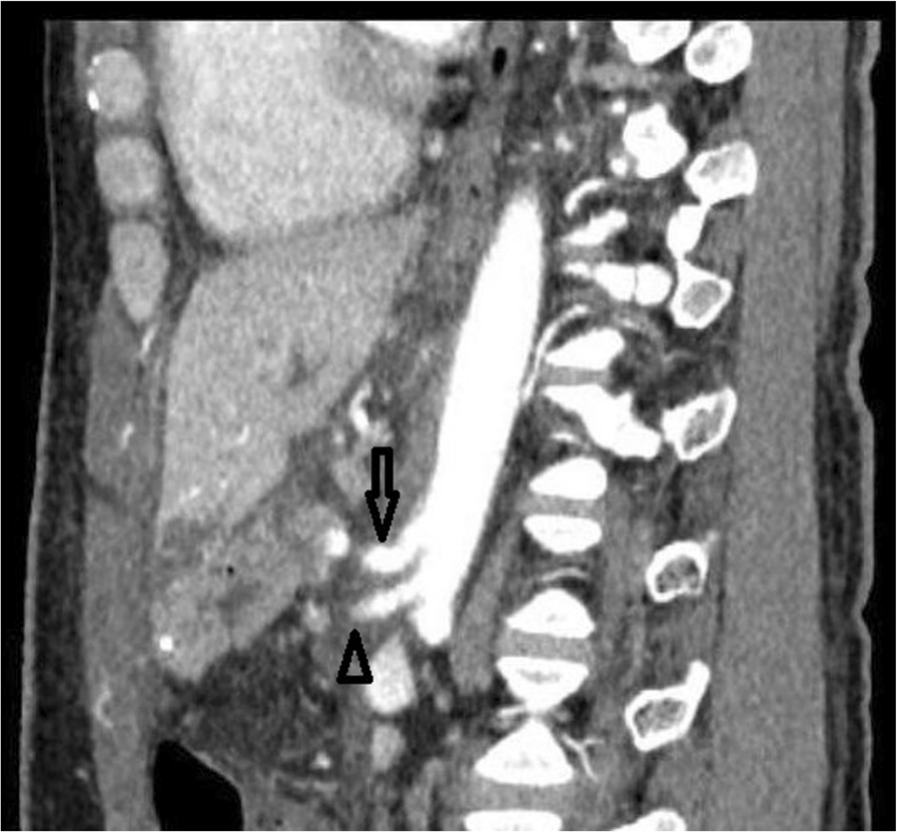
CT scan after the second operation showed that the stenosis of celiac axis was fully released (the *arrow* shows the CA and the *arrow head* shows the SMA)
